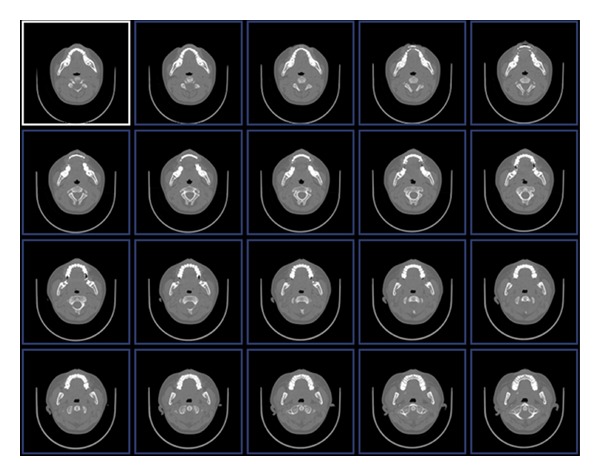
Part of the organized CT images in the top view.
